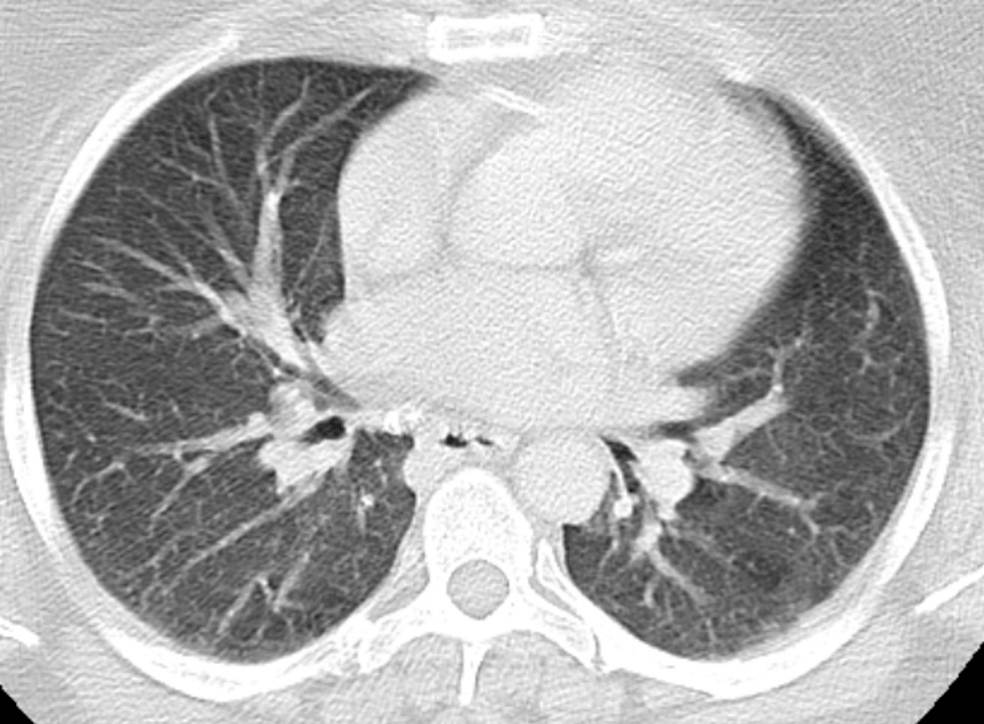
Repeat CT after resection of the mass showing no radiographic disease
